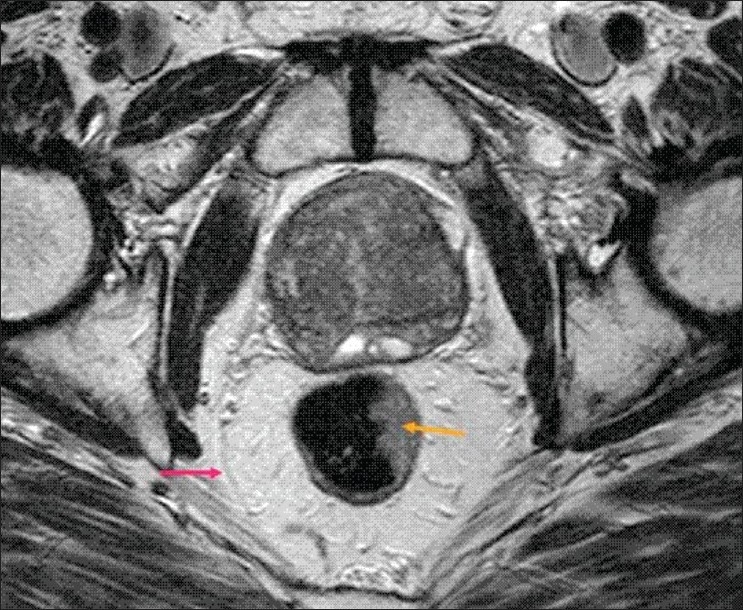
MRI echo T2 w spin echo image shows CRM - peritoneal reflection (arrow pink). Note the eccentric left lateral wall tumour (yellow arrow). Note the CRM is not involved in this case
